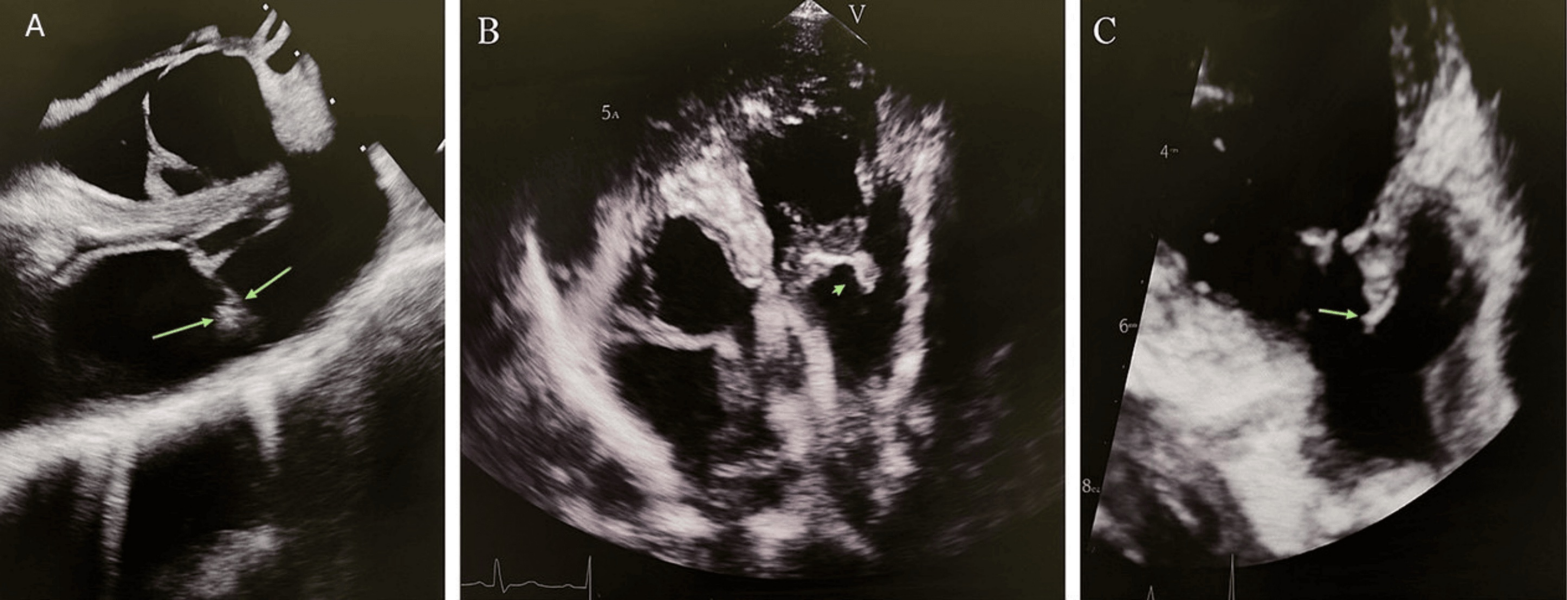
TEE showing a large, mobile, echodense structure measuring 0.7×1.22 cm attached to the anterior leaflet of the tricuspid valve, consistent with tricuspid vegetation (green arrows). The images show (A) parasternal long-axis view, (B) apical four-chamber view, and (C) transesophageal echocardiography mid-esophageal view (four chamber). TEE: transesophageal echocardiogram
